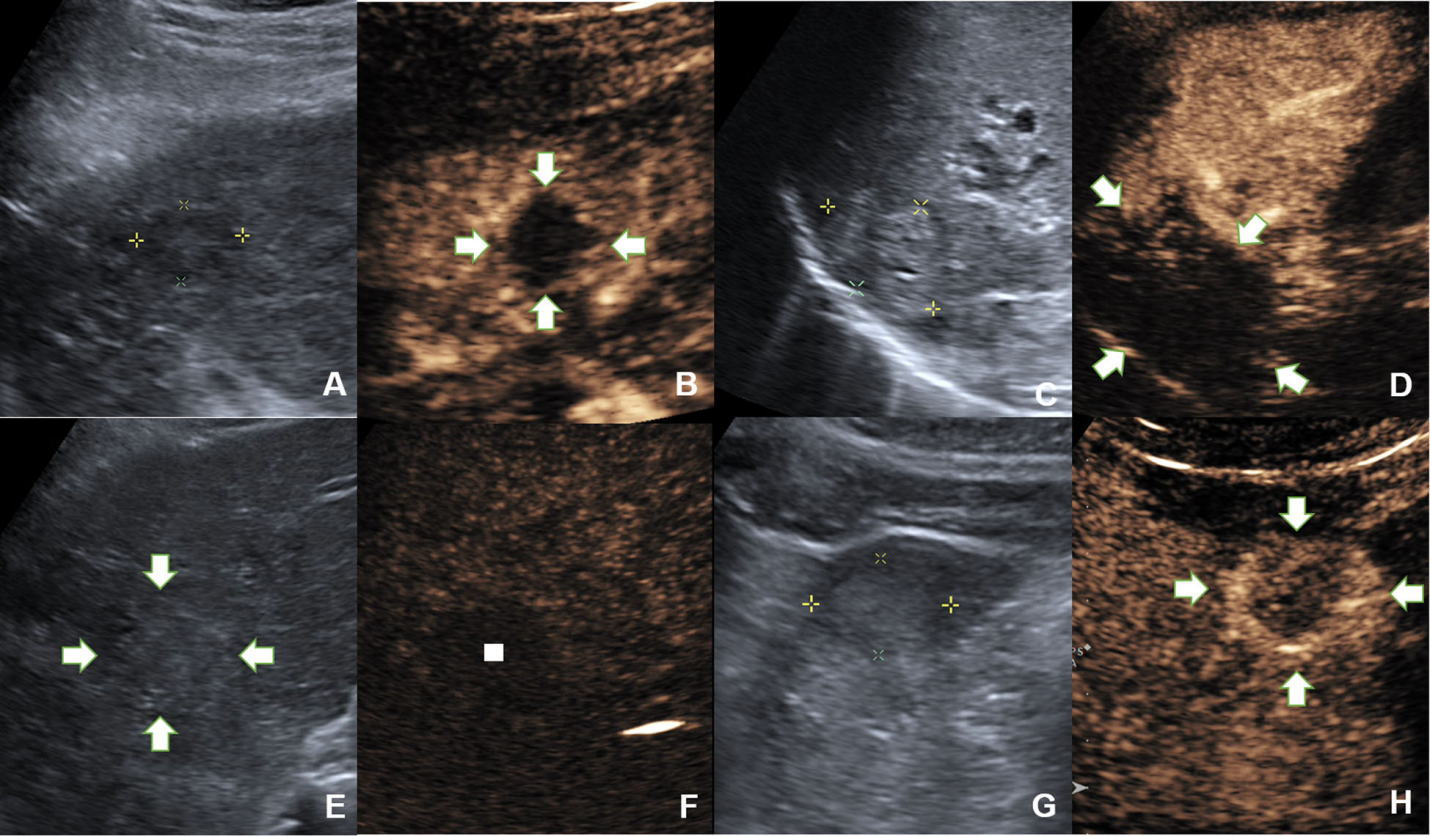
Examples of image evaluation in IA-CEUS. A & B, A hypoechoic lesion without intratumoural enhancement in SMA-CEUS preoperatively, the dimension of non-enhancing area (indicated by the arrow) was equal to the size in B-mode ultrasound. C & D, A hyperechoic lesion without intratumoural enhancement in SMA-CEUS preoperatively, the dimension of non-enhancing area (indicated by the arrow) was larger than the size in B-mode ultrasound. E & F, In preoperative HA-CEUS, there was no corona enhancement around the tumour (indicated by the arrow in B-mode and by rectangle in HA-CEUS) in late enhancing phase. G & H, In preoperative HA-CEUS, corona enhancement can be seen around the tumour (indicated by the arrow) in late enhancing phase. HA-CEUS = hepatic artery contrast-enhanced ultrasound; SMA-CEUS = superior mesenteric artery contrast-enhanced ultrasound
